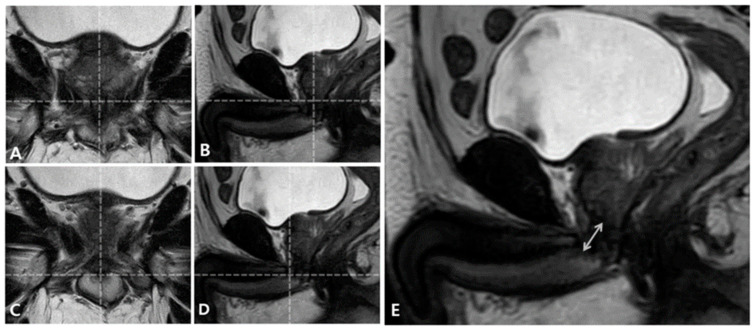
The membranous urethral length (MUL) measurement in prostate MRI. MUL is measured using a 3-axis cross-reference tool on prostate MRI and is defined as the distance from the superior border of the penile bulb to the inferior border of the prostate apex. (A,B) Identification of the inferior border of the prostate apex in the coronal and mid-sagittal planes. (C,D) Identification of the superior border of the penile bulb in the coronal and midsagittal planes. (E) Visual representation of the complete MUL trajectory across imaging planes. The white double-headed arrow indicates the measured membranous urethral length (MUL).
